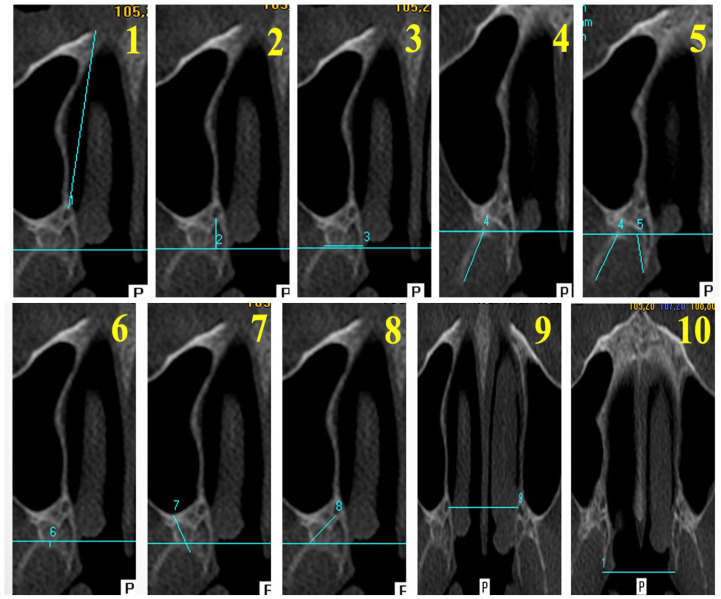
Axial CBCT measurements of the evaluated parameters. (1) AL—Anterior Length; (2) PL—Posterior Length; (3) PPW—Pterygoid Process Width; (4) PPT—Pterygoid Process Thickness; (5) LLL—Lateral Lamina Length; (6) MLL—Medial Lamina Length; (7) PMT—Pterygomaxillary Thickness; (8) DF–GPC—Distance between the Pterygomaxillary Fissure and the Greater Palatine Canal; (9) DGPC—Distance Between Greater Palatine Canals; (10) DML—Distance Between Medial Lamina Endpoints.
